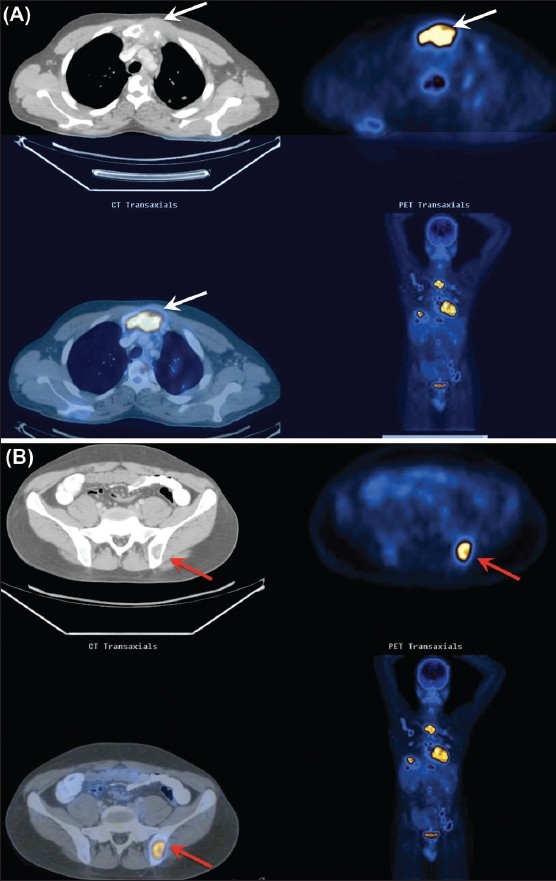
FDG-PET/CT of the chest (A) demonstrates metabolically active sternal (white arrows), vertebral body, and scapular lesions, as well as metabolically active left lung nodules. Biopsy from the sternal lesion showed tuberculosis. FDG-PET/CT (B) of the pelvis demonstrates a metabolically active osteolytic lesion in the left ilium (red arrows). Biopsy from this lesion showed tuberculosis as well
